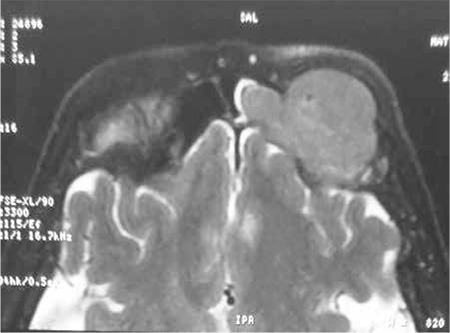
MRI images displaying the mass with extension into the orbit.
